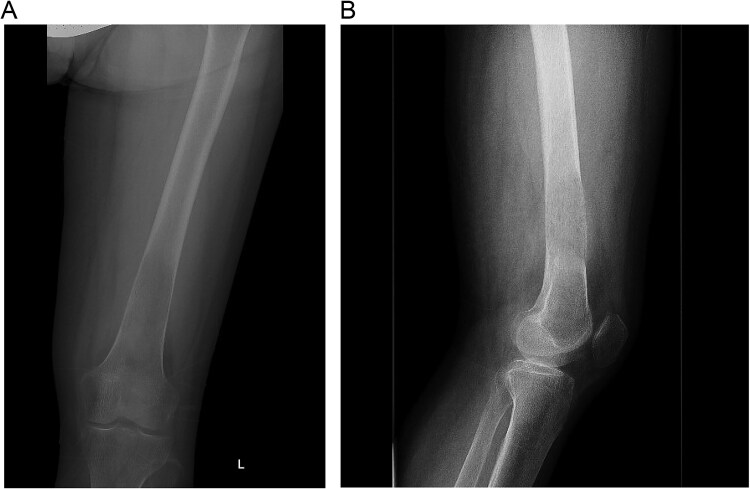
(A and B) Initial radiographs of the distal femur lesion.
